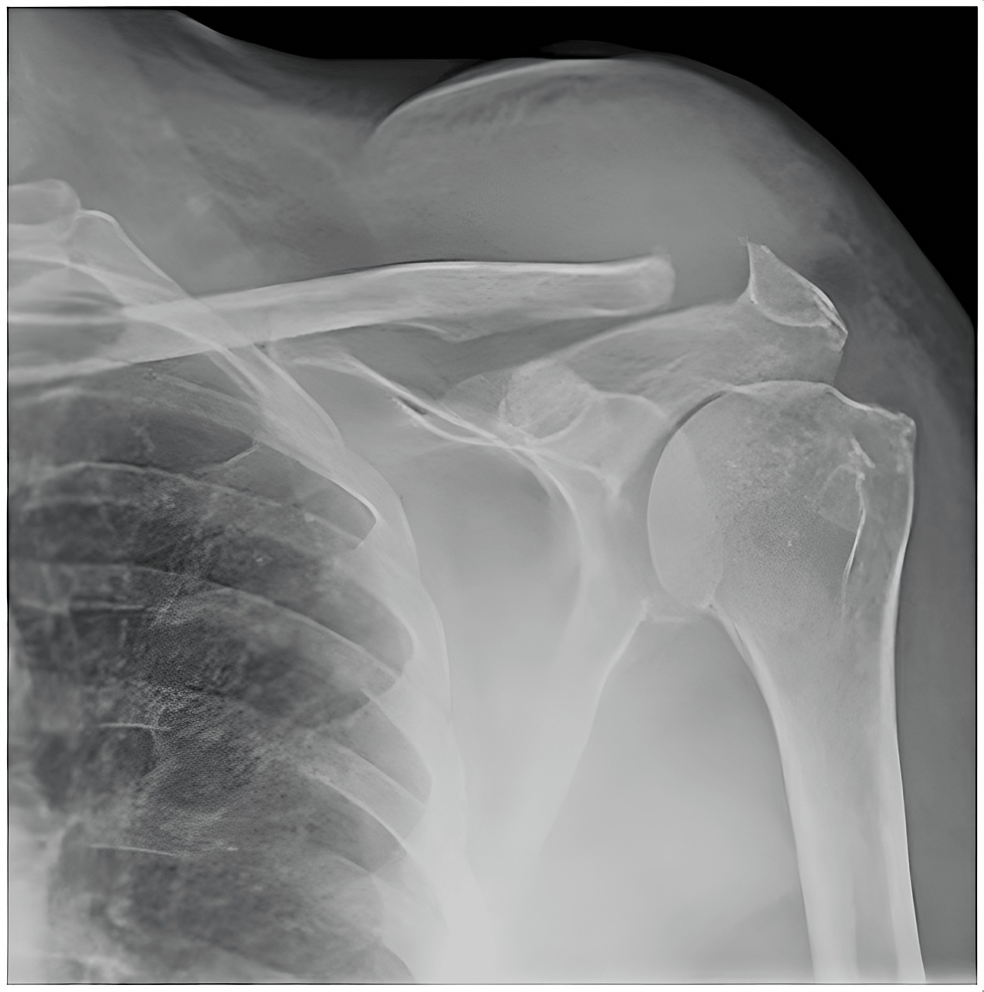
X-ray left shoulder Increased soft tissue shadow above the acromioclavicular joint and widening of the joint
